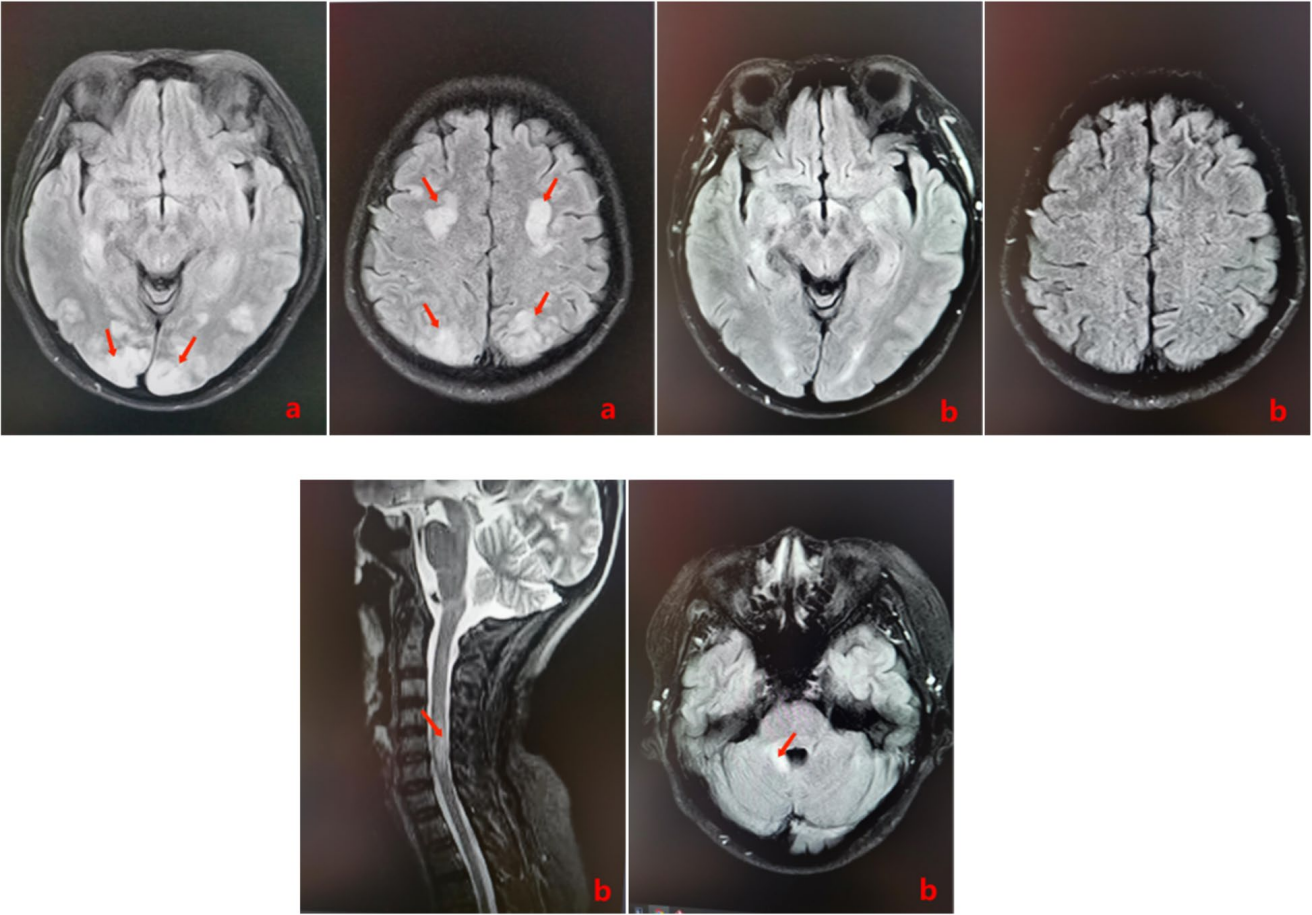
a MRI shows that bilateral frontal, occipital,and parietal new-onset brain gyrus edematous lesions (April 27, 2022). b MRI shows the disappearance of bilateral frontal, occipital,and parietal new-onset brain gyrus edematous lesions and residual lesions of the focal lesions at the dorsal pons near right surface of the fourth ventricle and spinal cord of the C4-C6 vertebrae
